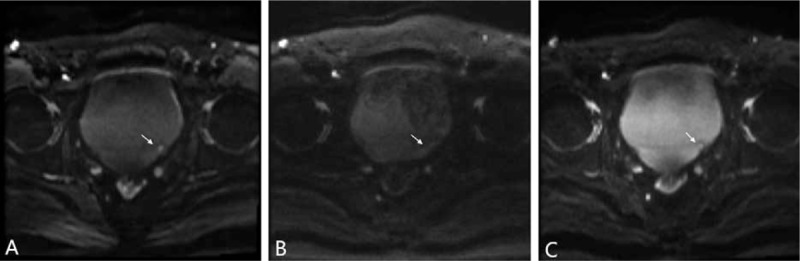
Comparison of (A) iShim, (B) RESOLVE and (C) ss-EPI DWI images (b = 1000 sec/mm^2^) in a 50-year-old male patient with single bladder cancer located in posterior wall (arrow). The size of the lesion is 0.4 cm, which is more clearly visible on (A) iShim DWI compare with (B) RESOLVE and (C) ss-EPI. Confidence score on (A) iShim, (B) RESOLVE and (C) ss-EPI is 3,1 and 2. DWI = diffusion-weighted imaging, iShim = integrated slice-specific dynamic shimming, RESOLVE = readout segmentation of long variable echo trains, ss-EPI = single-shot echo-planar-imaging.
